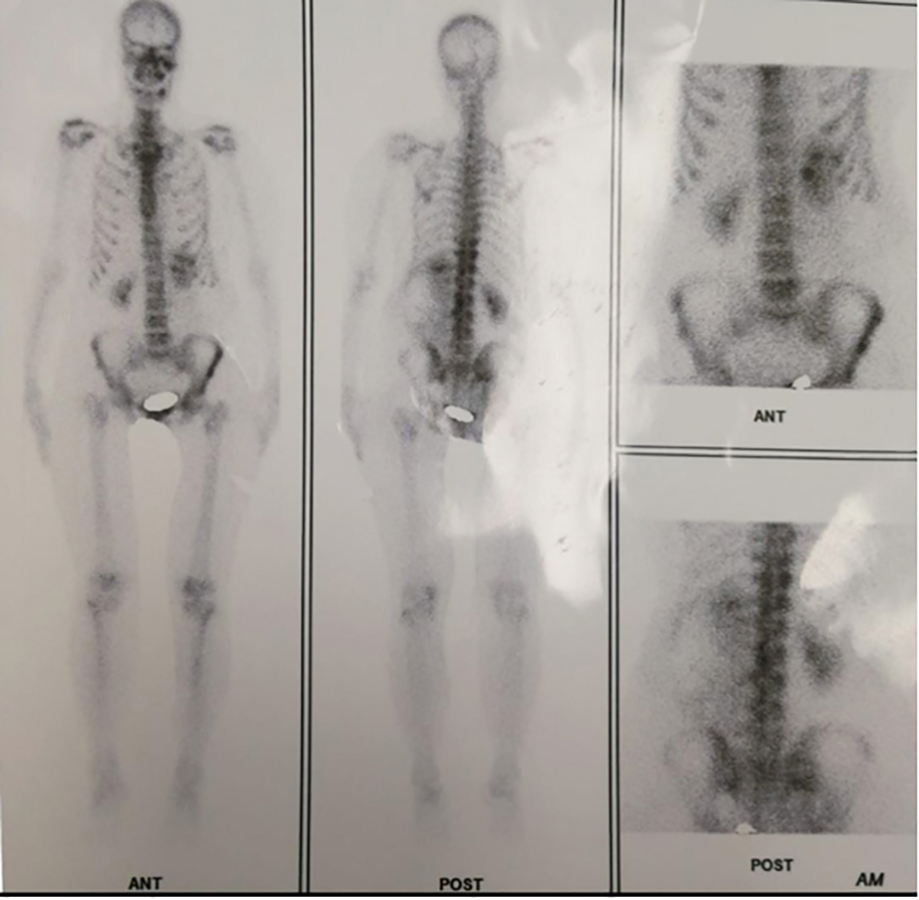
PET CT scan findings favouring soft tissue mass in left paraspinal region causing erosion of the 12th rib and adjoining transverse process of L1-2 vertebra.
